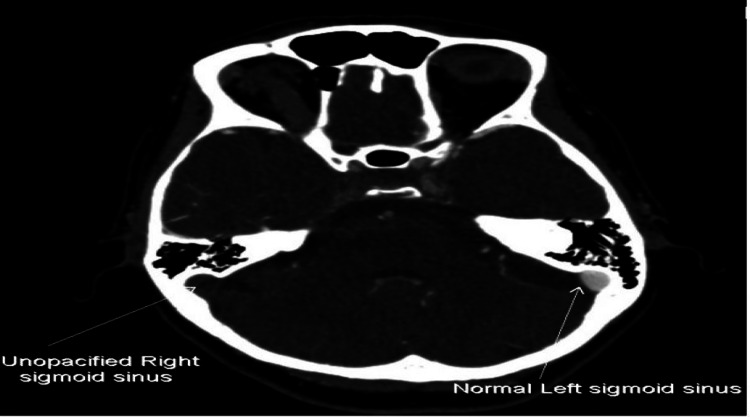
Axial view of the CT cerebral Venogram demonstrates non-enhancement of the thrombosed Right Sigmoid sinus.
